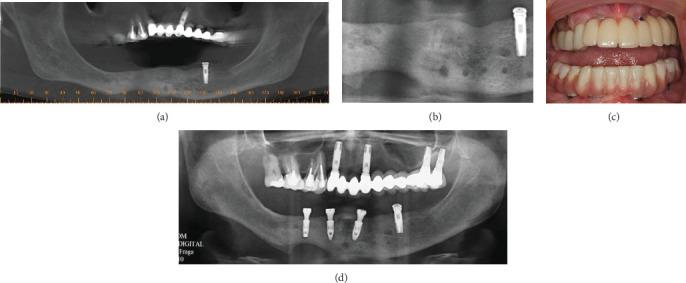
(a, b) CBCT scan shows bone remodeling in the area of osteomyelitis. (c, d) Implant follow-up and prosthetic rehabilitation 2 years later.
